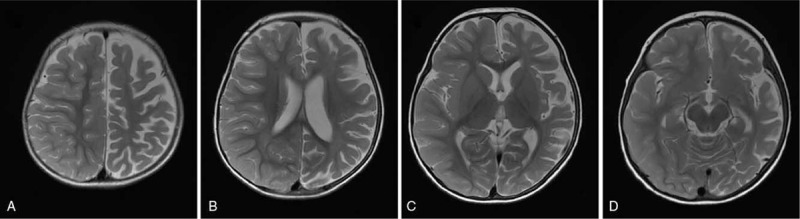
MRI findings 12 months after the onset. A-D: T2-weighted MRI showed atrophy of the left hemisphere.
